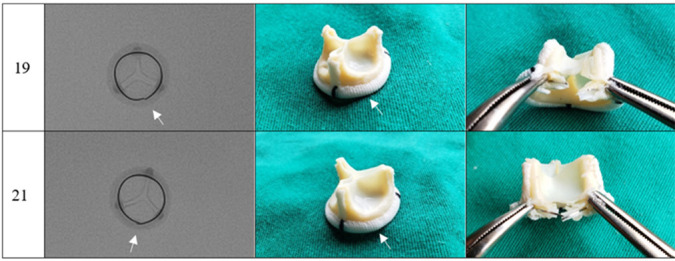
Braile prosthesis after fracture, sizes 19 mm and 21 mm. Radiography (superior-inferior projection) and photographs (intact lining and dissected lining). Arrow corresponding to the fracture site.
